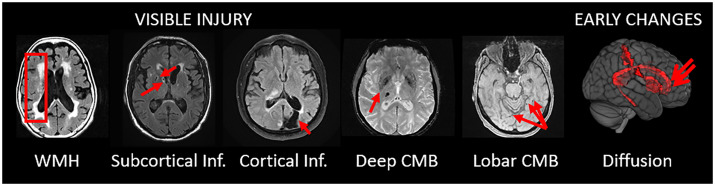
Measured CVD-related changes on MRI. The visible CVD injury/lesions are showed on the left and the tracts where early CVD related changes are measurable on diffusion MRI are shown to the far right.
